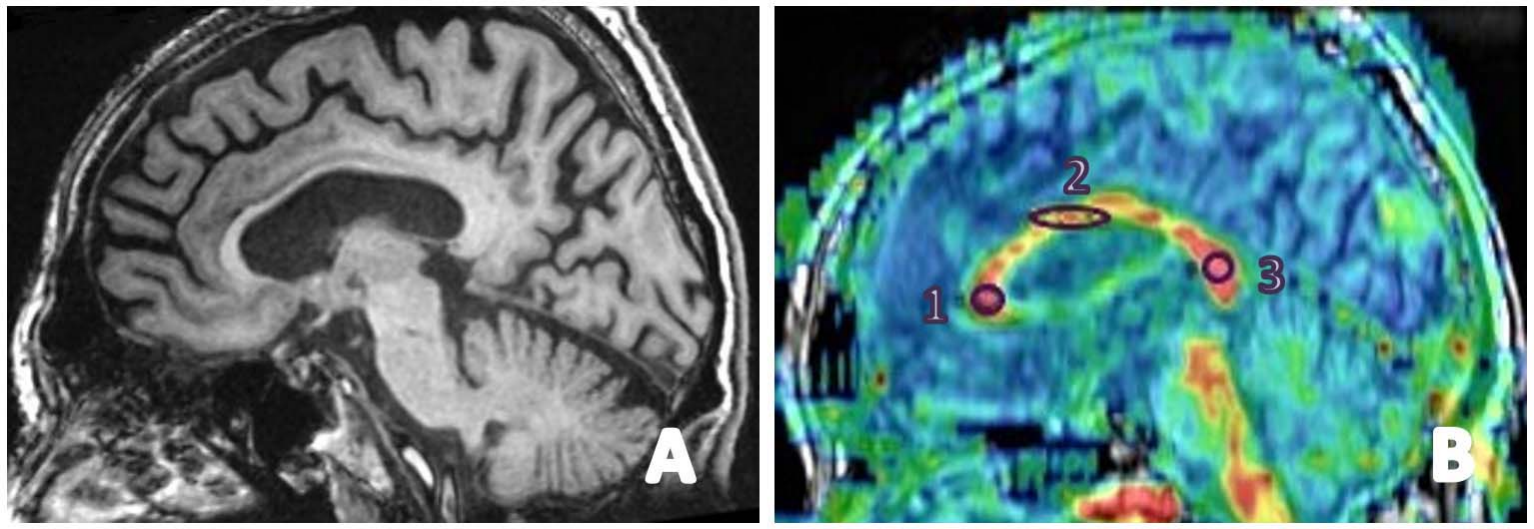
The figure shows fractional anisotropy of the corpus callosum in a male patient with clinical presentation of Lewy body dementia. (A) A T1W image in the sagittal plane; (B) a fusion image of T1W and color-coded fractional anisotropy maps made on the General Electronics 3T MRI workstation. Fractional anisotropy (FA) of the corpus callosum with region of interest put on: 1) genu with FA ═ 0.72, 2) corpus with FA ═ 0.50, and 3) splenium with FA ═ 0.85. MRI: Magnetic resonance imaging.
